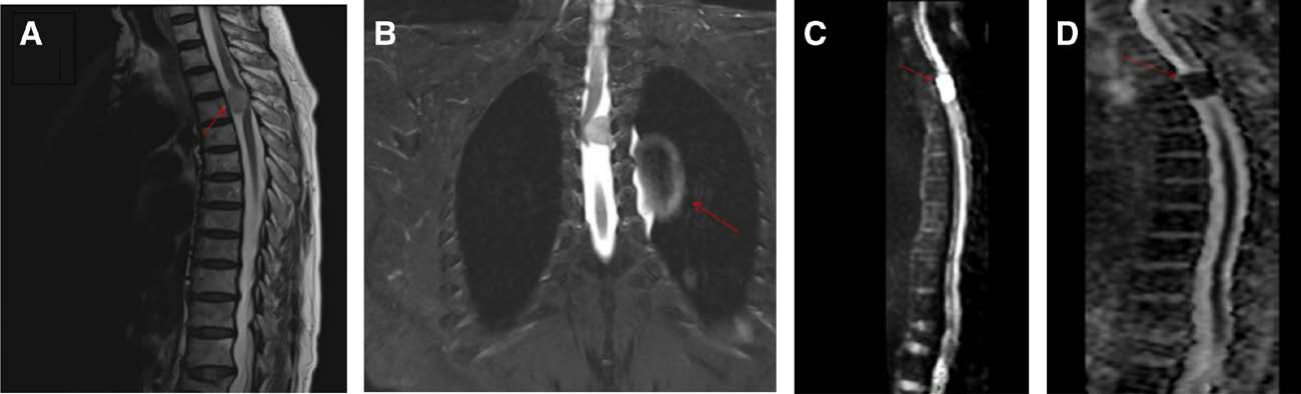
Preoperative imaging: (A) preoperative sagittal MRI; (B) preoperative coronal MRI; (C) preoperative sagittal enhanced MRI; (D) preoperative enhanced MRI. Soft tissue signal nodular shadow was seen on the left side of the spinal canal at the level of the thoracic 3 to 4 vertebral body, showing T1WI iso-signal, DWI high signal, ADC low signal, size about 14 mm × 20 mm × 12 mm, adjacent to the spinal cord compression shifted to the right, the lesion localized. The lesion appeared to be connected to the wide base of the left dura mater, and showed significant uniform enhancement after enhancement. The diagnosis is a left-sided occupying lesion at the level of the thoracic 3 to 4 vertebral body: suggesting a possible spinal meningioma. MRI = magnetic resonance imaging.
